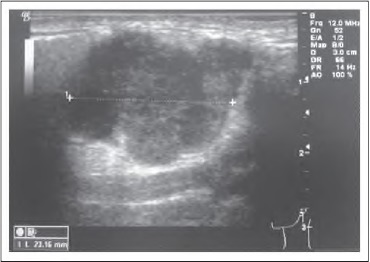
Ultrasonography measurement using a Philips iU22 xMATRIX ultrasonography machine
